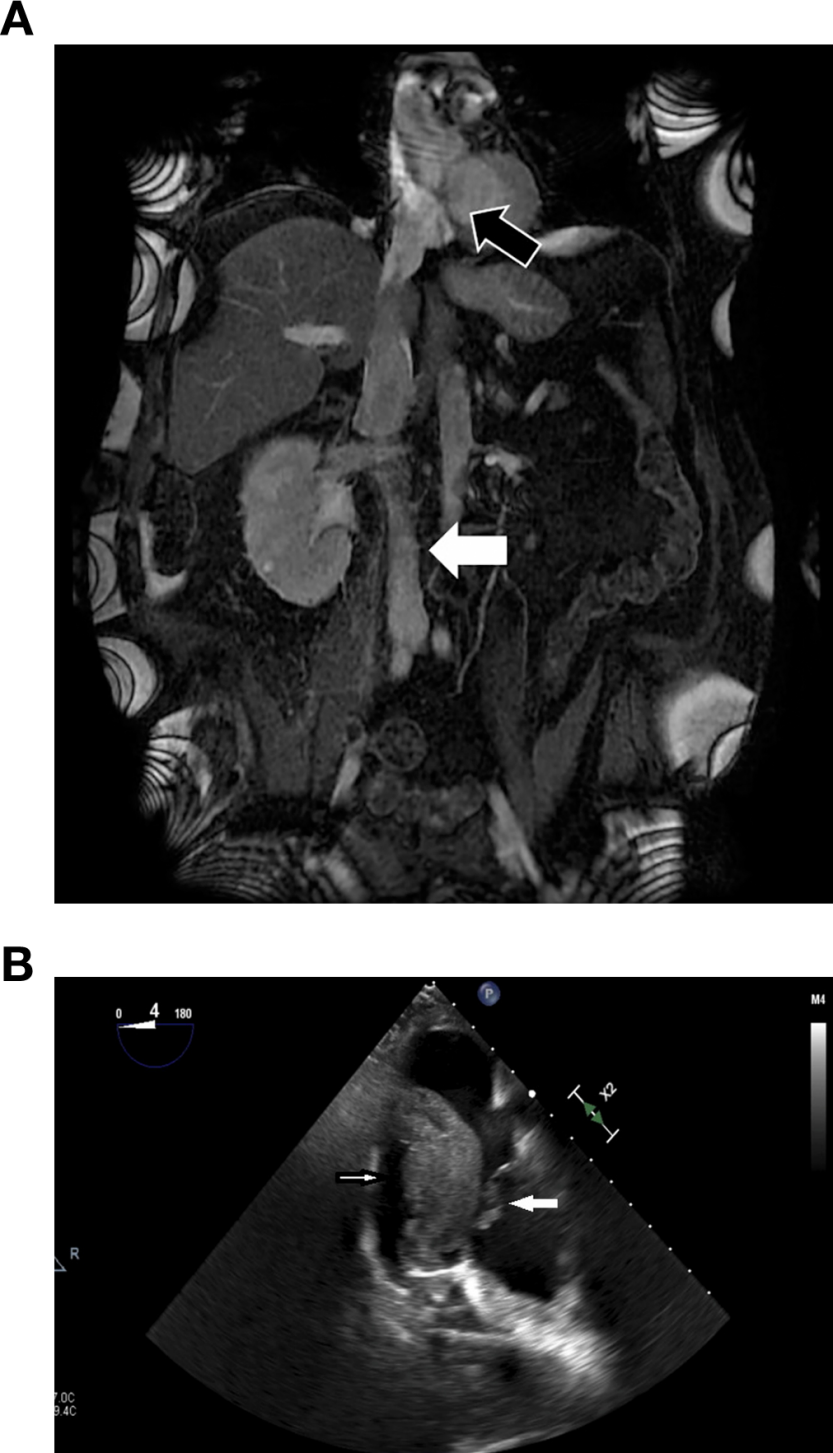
(A) Magnetic resonance imaging showing a right kidney tumor and the inferior vena cava (IVC) full of tumor thrombus (TT) all the way to the right atrium (black arrow) and blood thrombus below the TT (white arrow). (B) Four-chamber transesophageal echocardiography view showed a segment of TT migrated to RA (small white arrow) and bouncing on tricuspid valve (big white arrow).
